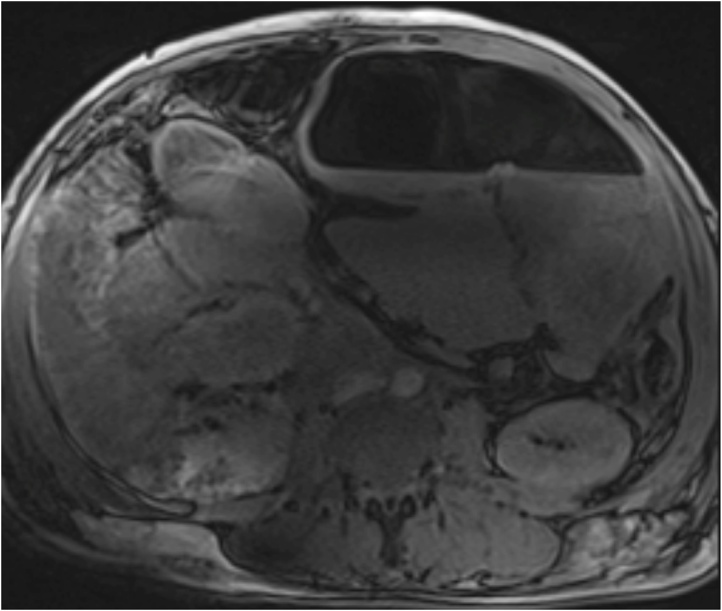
MRI abdomen, axial view showing large bowel volvulus in the left upper quadrant.
